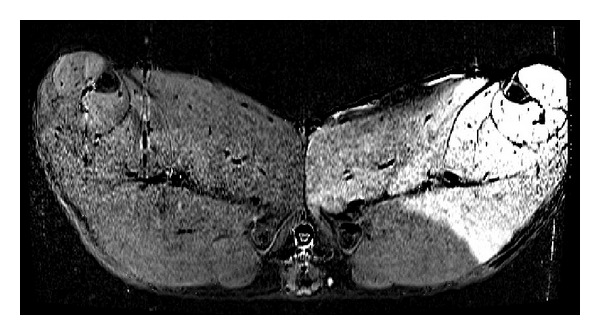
Difference image. T1-weighted images before and after local injection of contrast agent into the right femoral artery were acquired. There is significant brightening of the supply area.
